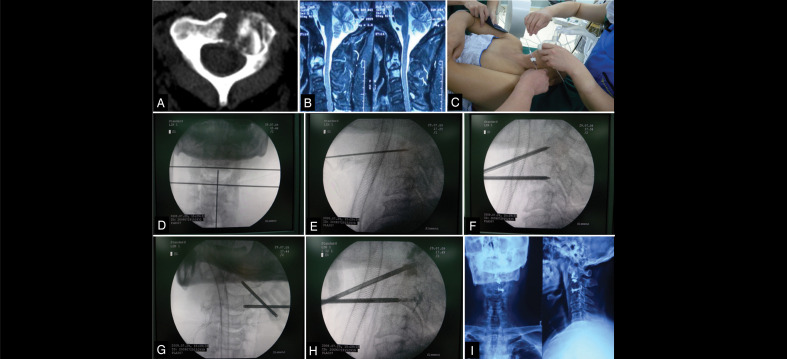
Preoperative CT showing lytic destruction of the vertebral body. B: Preoperative MRI demonstrating tumor invasion and signal changes in C2 and C3 vertebral bodies. C and D: Surface localization of the pedicle awl, determining the incision site. E-H: Fluoroscopic-guided simultaneous positioning, puncture, and bone cement infusion of the pedicle awl at C2 and C3. I: Postoperative X-ray showing satisfactory distribution of bone cement.
